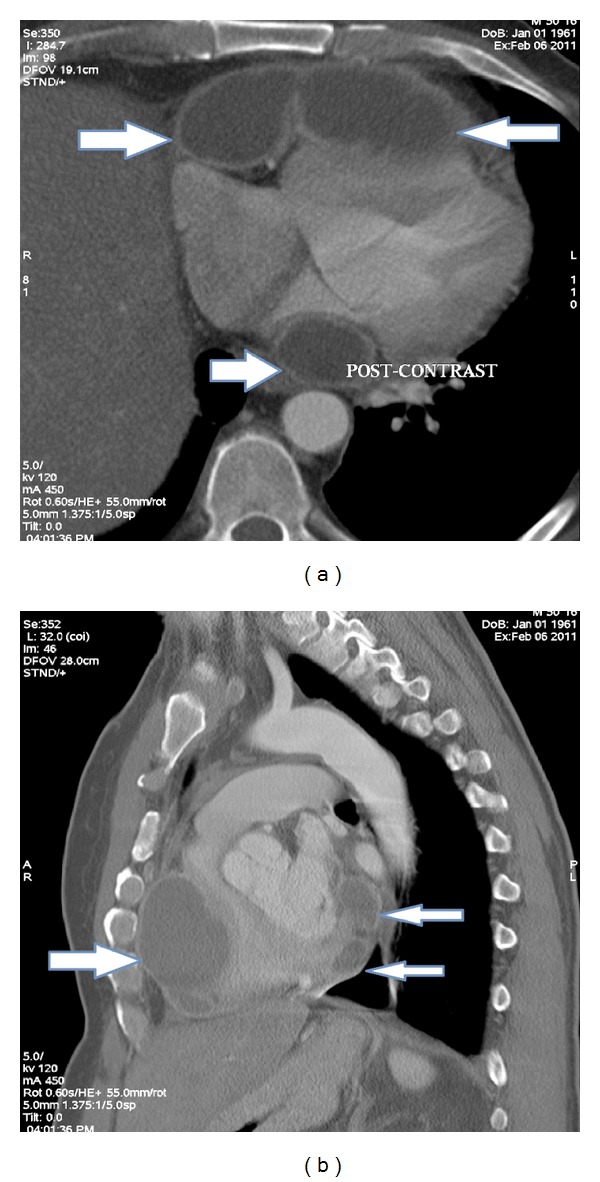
CT scan of chest shows the cysts compressing the right ventricle anteriorly (large arrows) and the cyst behind the heart compressing the left atrium posteriorly (small arrows).
